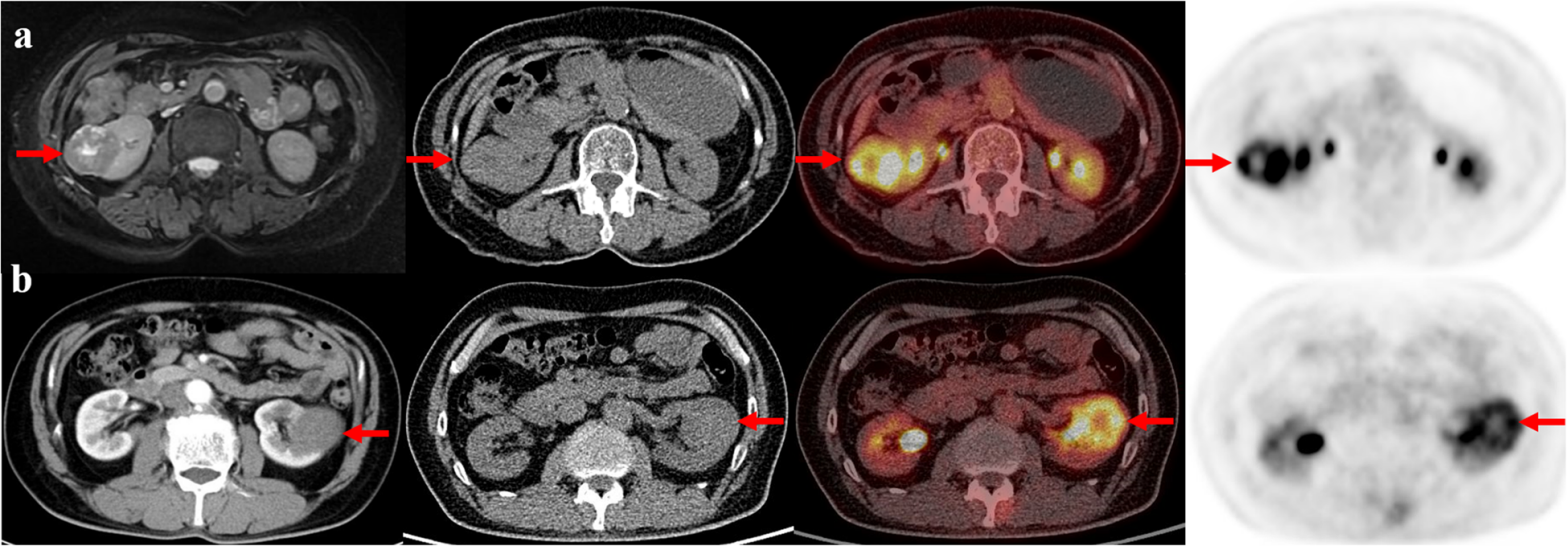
Representative cases. a The axial images from left-to-right are MR, coregistered CT, PET/CT fusion and PET. A 63-year-old woman with type 2 papillary renal cell carcinoma (WHO G3) in the right kidney (arrows). The primary tumor size is 4.4 cm, and the tumor SUVmax is 10.4. b The axial images from left-to-right are diagnostic contrast-enhanced CT, coregistered CT, PET/CT fusion and PET. A 61-year-old man with type 1 papillary renal cell carcinoma (WHO G2) in the left kidney (arrows). The primary tumor size is 4.2 cm, and the tumor SUVmax is 6
